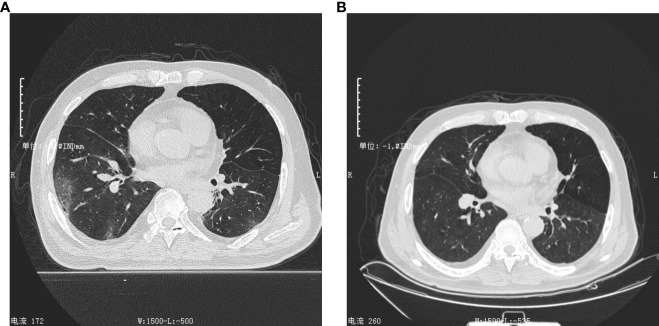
Lung computed tomography (CT) scan. (A) CT scan of the first admission on December 20, 2022. This image showed emphysema and multiple ground-glass image changed in both lungs. (B) CT scan of the second admission on February 22, 2023. This image showed emphysema, and multiple ground-glass opacities in both lungs were obviously absorbed.
